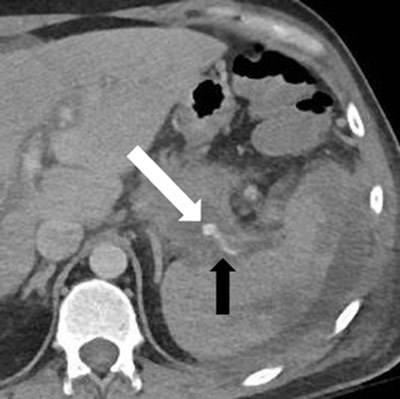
Enhanced CT, a few days later: recurrent hemorrhage (white arrow) arising retrogradely from the distal splenic artery (black arrow).
